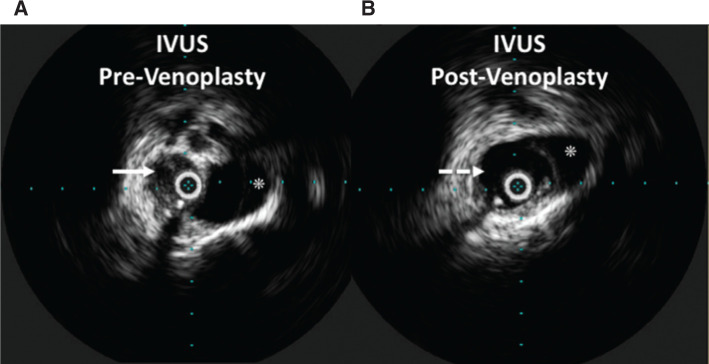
Intravascular ultrasound images of the SVC were created immediately post–lead extraction (A) and following serial venoplasty (B). The immediate post–lead extraction image is notable for a stenotic SVC with intraluminal thrombus (solid arrow). Following three serial venoplasty balloon dilations, the SVC was noted to be expanded (B). The azygos vein is visualized in both images (see asterisk).
